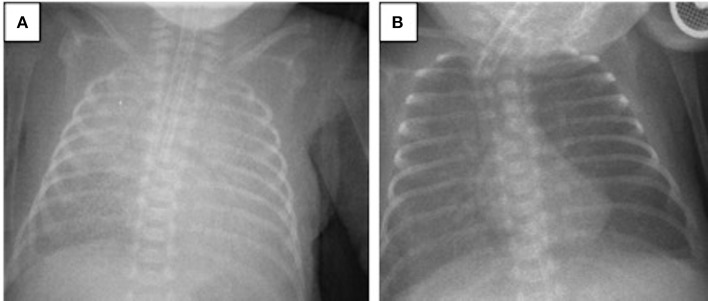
Chest radiograms of Case 2: (A) At the time of ARDS diagnosis, an X-ray revealed bilateral irregular diffuse opacity; (B) improvement in lung aeration was seen after one dose of surfactant plus budesonide treatment.
